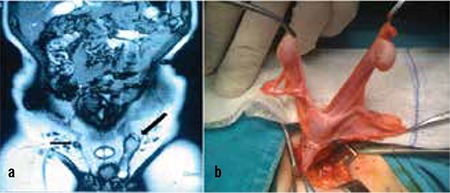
MR images of both inguinal canals, showing right atrophic and left normal testes located in the corresponding inguinal canalb) Intraoperative view of the testes, uterus and fallopian tubes in the left inguinal canal
